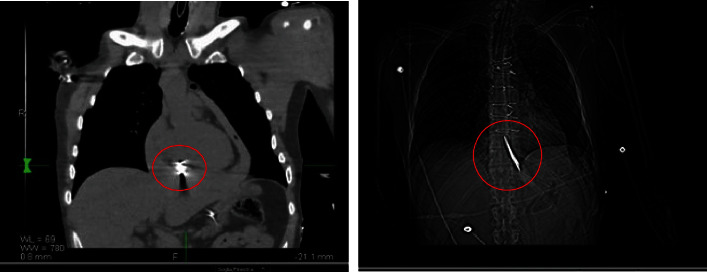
Frontal view of the chest on CT examination. The knife is represented by the red circle.
